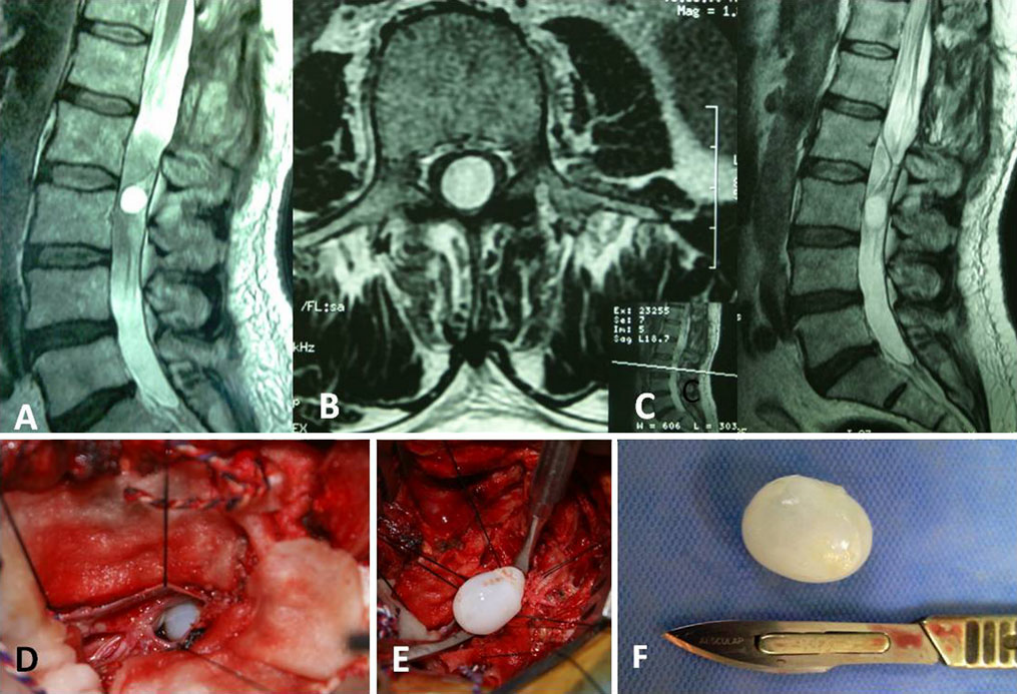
a–c On repeated MR imaging, a cystic structure was demonstrated at the L3 level, a level below the prior operated level. d–f After opening the dura, an intradural extramedullary cystic mass was determined. The lesion was removed gross totally
